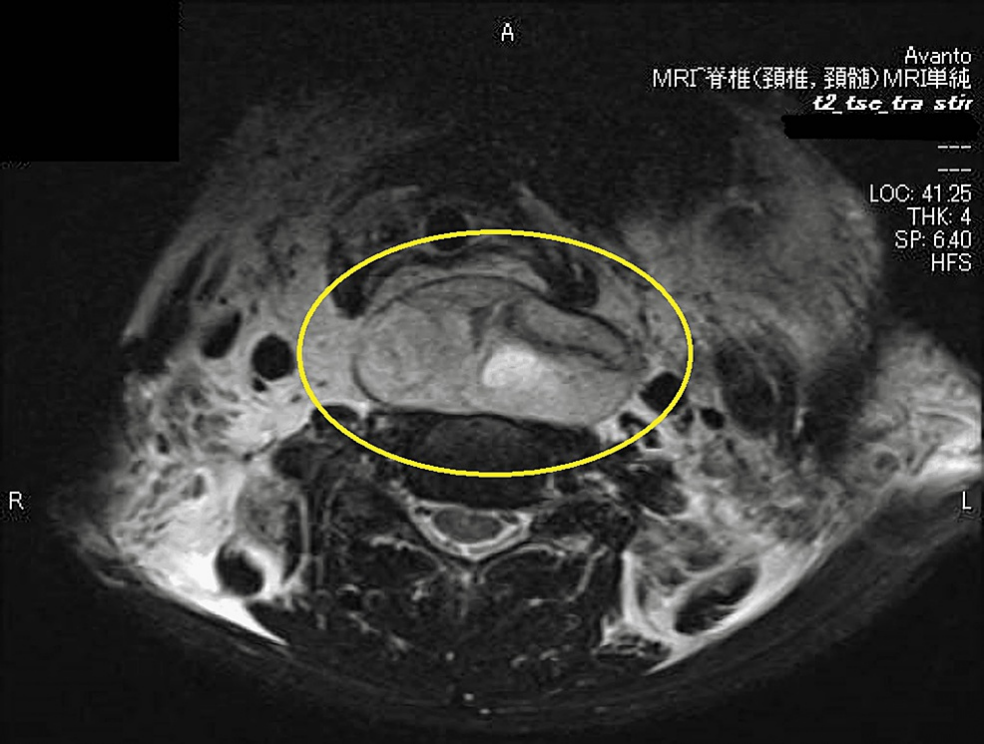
Cervical spine MRI at initial examination (axial) MRI also shows swelling of the retropharynx.
